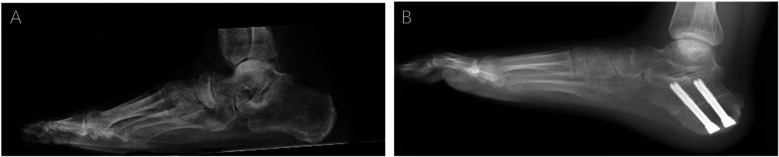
Weight-bearing lateral x-rays of the foot before and after ankle surgery. (A) Preoperative weight-bearing lateral x-ray of the foot: the preoperative lateral view shows significant impingement between the talus and the accessory anterolateral talar facet (AALTF), with abnormal alignment. (B) Postoperative image showing improved alignment and elimination of impingement after AALTF resection and flatfoot correction surgery.
